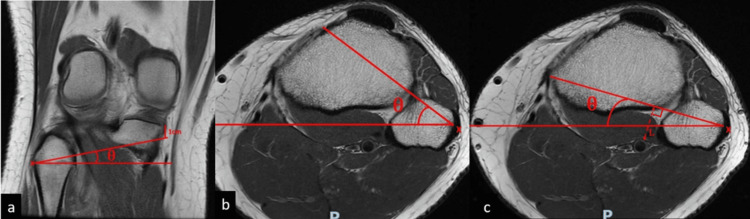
MRI imaging of the right knee in the coronal (a), and axial (b,c) views. The angles of superior, anterior, and posterior safe zones for PTFJ (θ), demarcated in red lines are measured against the horizontal. The superior safe zone for instrumentation was measured on the coronal cuts (a), to a pre-identified point 1 cm below the medial most aspect of the joint line. The anterior safe zone is measured on the axial cuts (b), to the medial most point of the tibial tubercle. The posterior safe zone is measured on the axial cuts (c), to the medial most point on the medial proximal tibia cortex. The nearest distance (L) between the posterior safe zone and the neurovascular bundle is measured.
